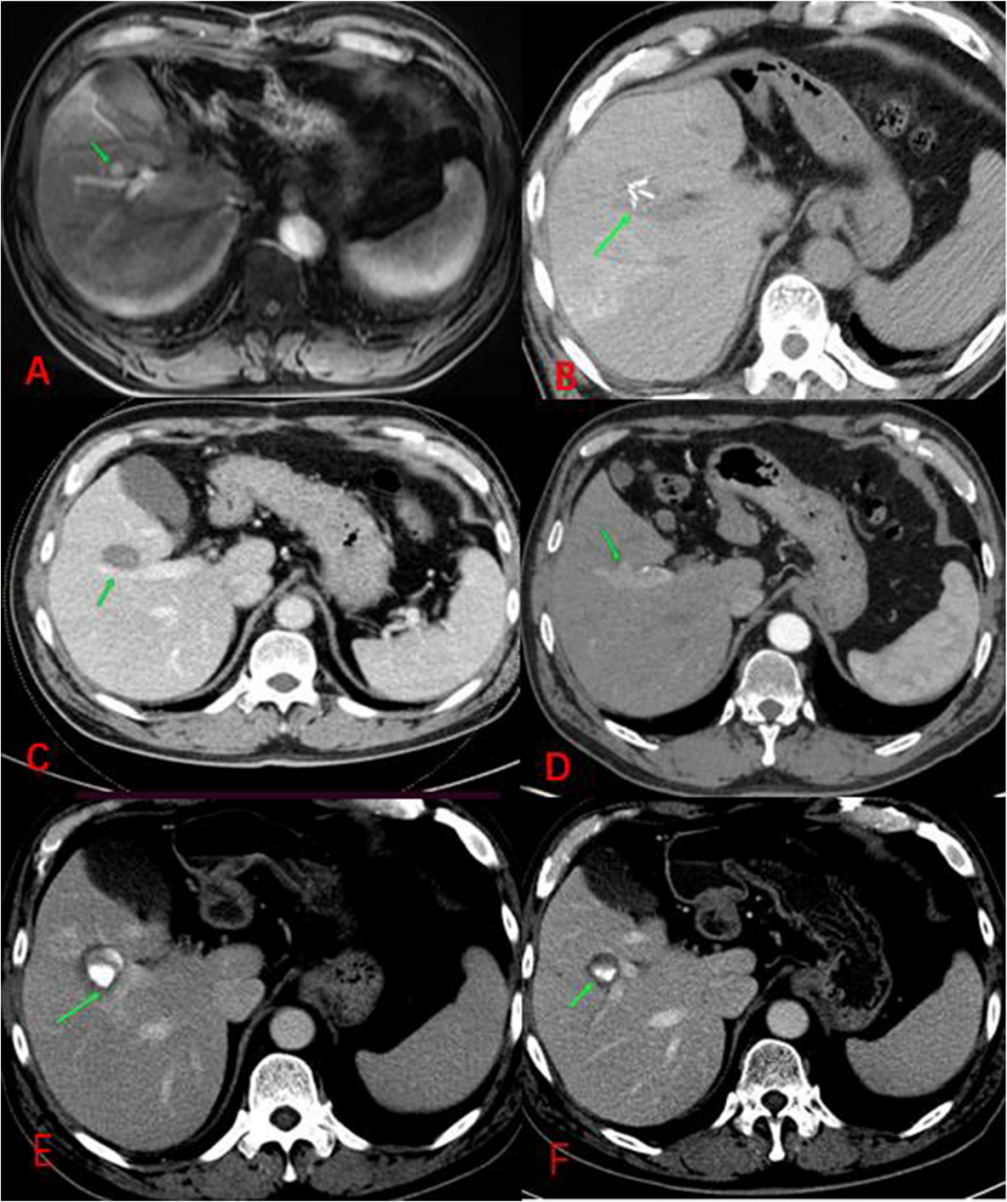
Local tumor progression (LTP) after RFA for periportal HCC in a 57-year-old man. (A) Dynamic contrast-enhanced axial magnetic resonance (DCE-MRI) scan obtained showing a small HCC (arrow) in periportal location before RFA. (B) CT scan obtained during RFA showing a multitip expandable electrode adjacent to the portal vein (arrow). (C) CT scan obtained during portal venous phase 1 months after RFA showing the complete ablation zone (arrow) adjacent to the portal vein. (D) CT scan obtained during hepatic arterial phase and portal venous phase (not shown) 53 months after RFA showing the LTP, a small arterial enhancing nodule (arrows), with washout at portal venous phase. (E) CT scan obtained during portal venous phase 1 months after TACE+RFA showing the complete ablation zone (arrow) adjacent to the portal vein, “intratumoral lipiodol deposition” can be seen in the ablation zone. (F) CT scan obtained during portal venous phase 4 months after TACE+RFA showing the complete ablation zone (arrow) adjacent to the portal vein
